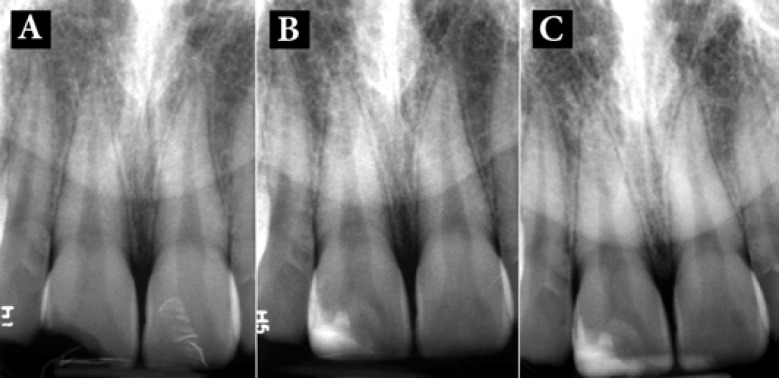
A) *Pre-operative periapical radiography of the maxillary right incisor with complicated crown fracture and pulp involvement; *B)* Post-operative periapical radiography after miniature pulpotomy and crown restoration; *C)* One-year follow-up; the tooth was vital, functional and symptom-free; note the thick dentinal bridge beneath CEM cement
